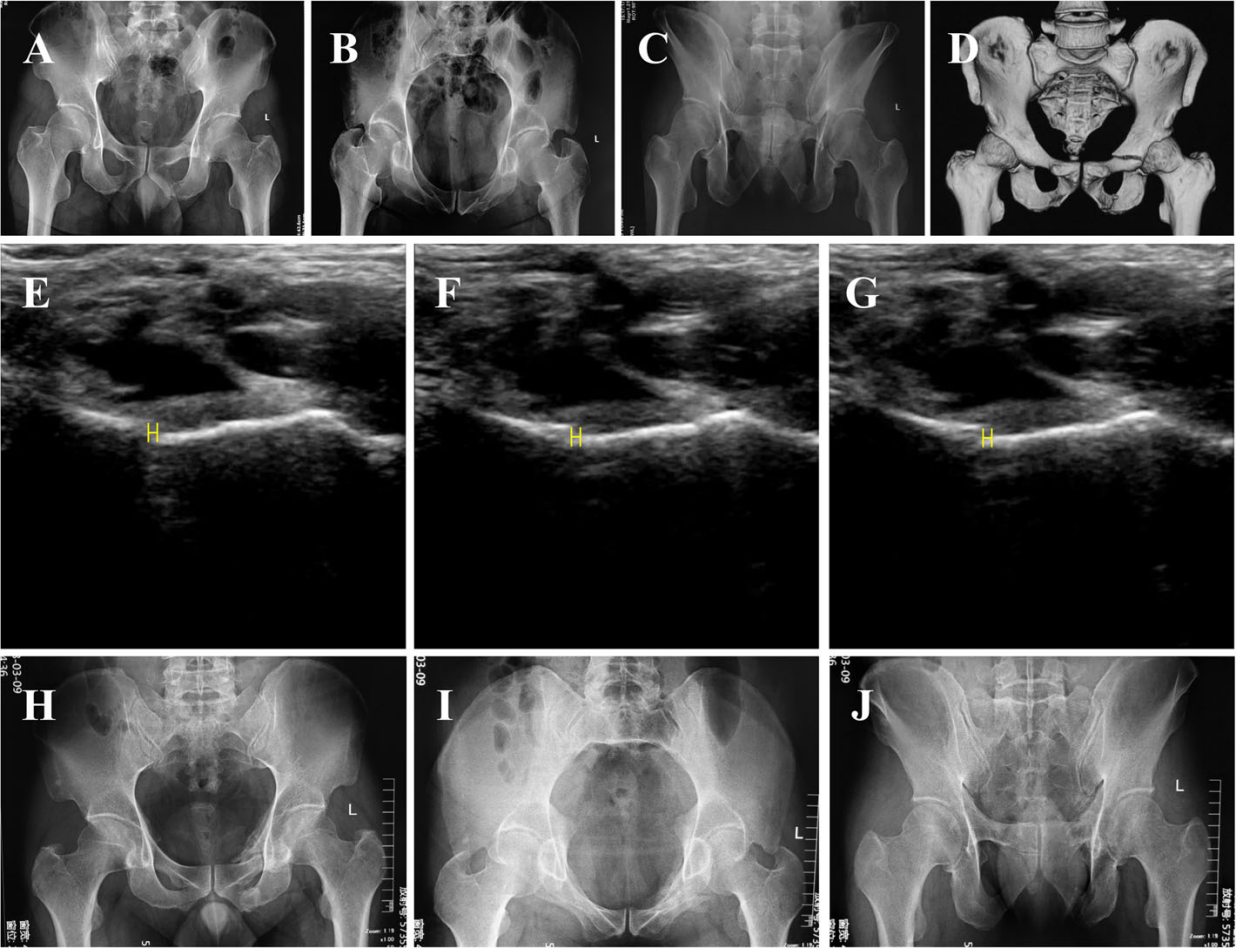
Case 2, 37-y-old male, suffered an accident. a-c Post-injury anterior-posterior, inlet and outlet X-rays; d Post-injury CT; e Displacement under ultrasonography when at rest; f Displacement under ultrasonography when compression; g Displacement under ultrasonography when separation. The left-right mobility was 0.03 cm and conservative treatment was chosen for the patient. h-j Post-injury 10 months anterior-posterior, inlet and outlet X-rays. The patient recovered well and Majeed score was 94.
